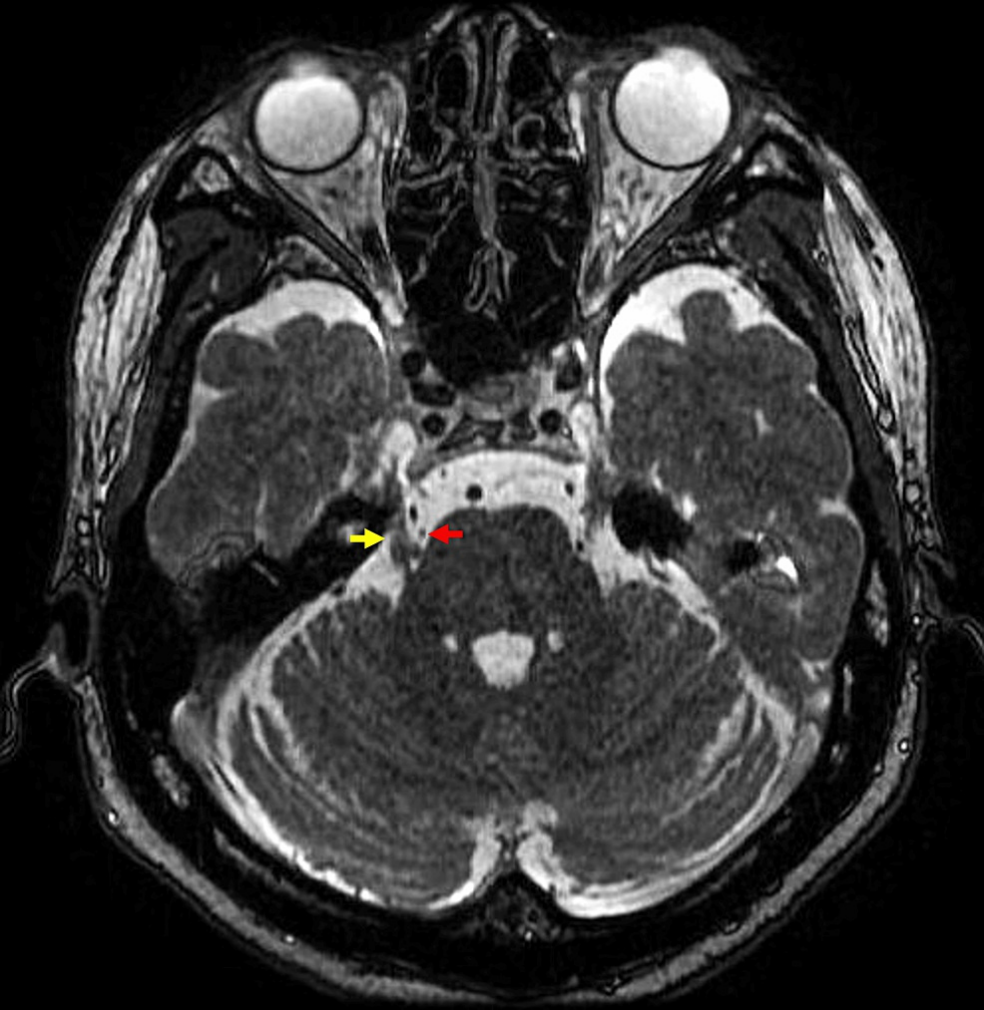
Horizontal section of MRI The arrowheads showed proximity of the superior cerebellar artery (red) and trigeminal nerve (yellow), but there was no evidence of neurovascular compression.
